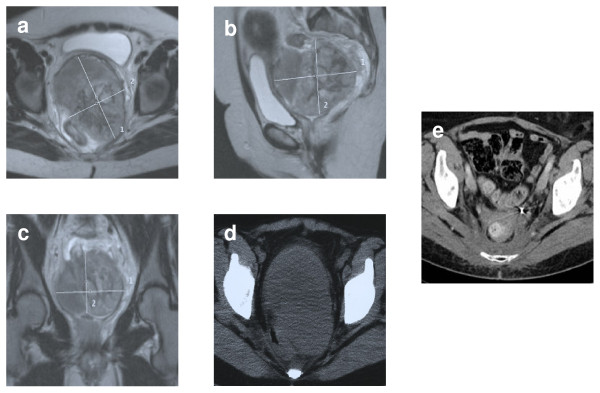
Case Report*. A 49-year-old female presented with obstipation, vaginal bleeding, urinary problems and chronic vaginal fluor. Assuming a gynaecological tumour a biopsy of the cervix was performed and showed a high grade pleomorphic sarcoma, NOS. The MRI revealed a 13 × 9 × 9 cm tumour between cervix, bladder and rectum (a-d). Due to the palliative intent a debulking of about 80% of the tumour was performed. Prior to radiooncological treatment a protective colostomy was performed. Radiochemotherapy to 60 Gy (hyperfractionated twice daily) was well tolerated, hyperthermia had to be discontinued after the first application due to circulation problems. The colostomy could be removed after therapy. The only relevant late effect of CTC grade III or higher was a unilateral ureteral stenosis treated with a Double-J-catheter on the respective side. Ten years after the therapy the patient was still free of disease (e).
